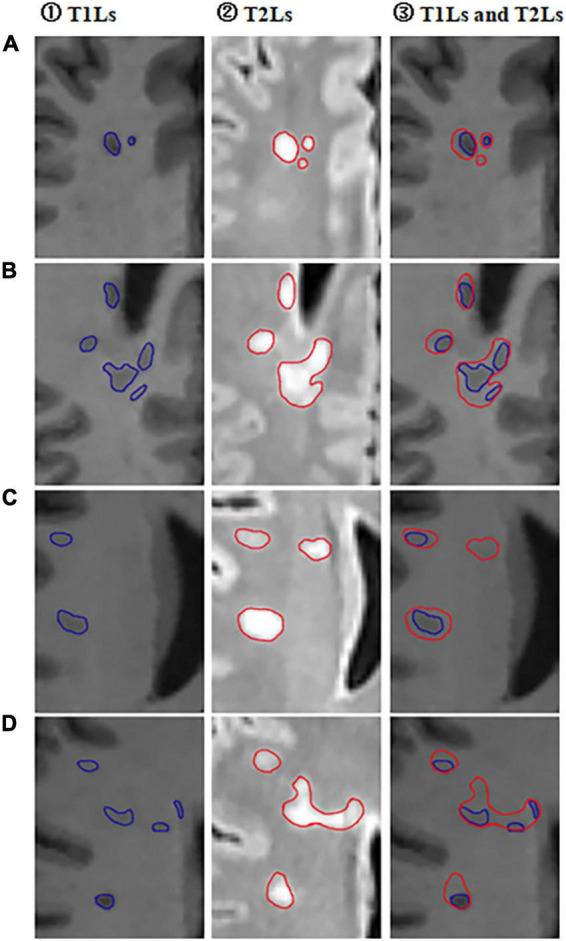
Selected partial axial images of MPRAGE and FLAIR sequences. (A–D) Partial axial FLAIR/MPRAGE images of four different RRMS patients in our cohort. ① Outlined T1-hypointense lesions (T1Ls) on MPRAGE images; ② Outlined T2-hyperintese (T2Ls) lesions on FLAIR images; ③ Outlined both T2-hyperintense lesions (T1Ls) and T1-hypointense lesions (T2Ls) on MPRAGE images.
